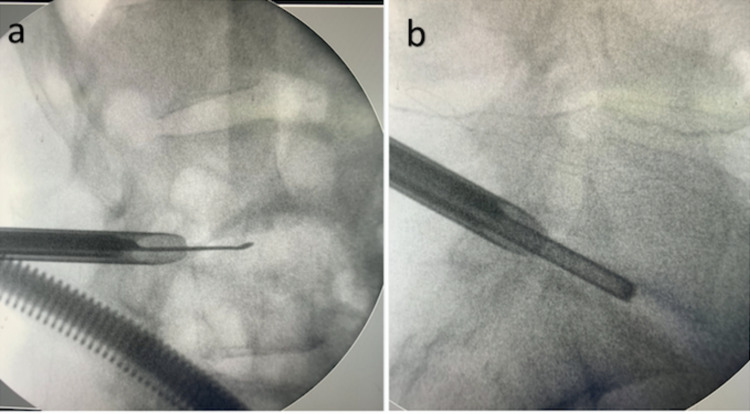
Lateral view of intraoperative X-ray of discs L2L3 (a) and L5S1 (b)
